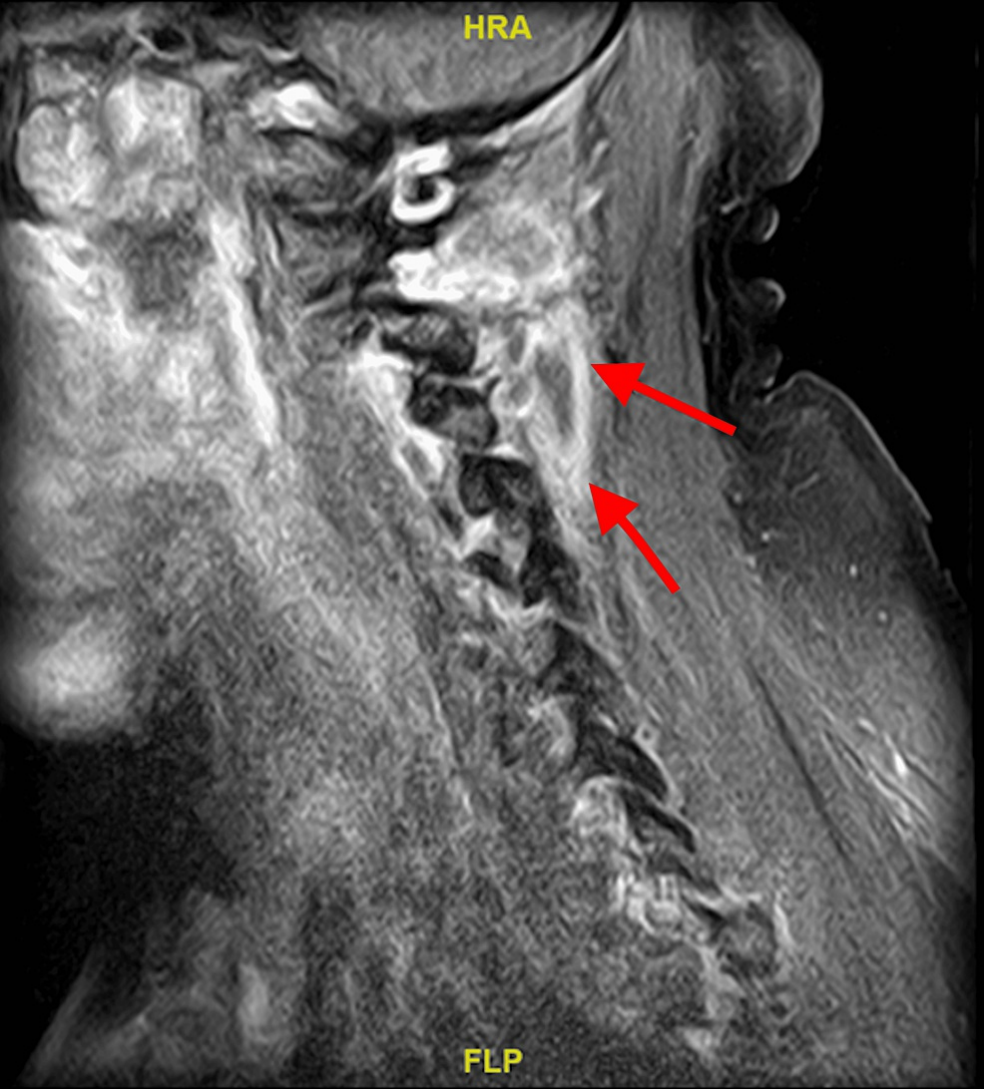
MRI with contrast of the patient's cervical spine showing a soft tissue lesion likely reflecting abscess with surrounding edema and enhancement (arrows)
